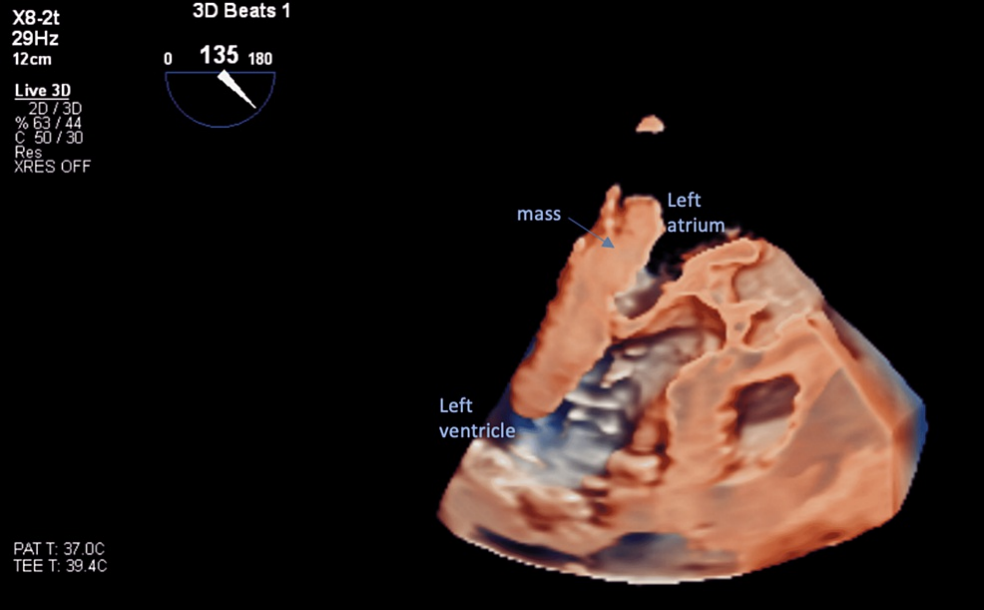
Three-dimensional transesophageal echocardiogram with mid-esophageal aortic valve long-axis view revealed the left atrial mass prolapsing into the left ventricle
